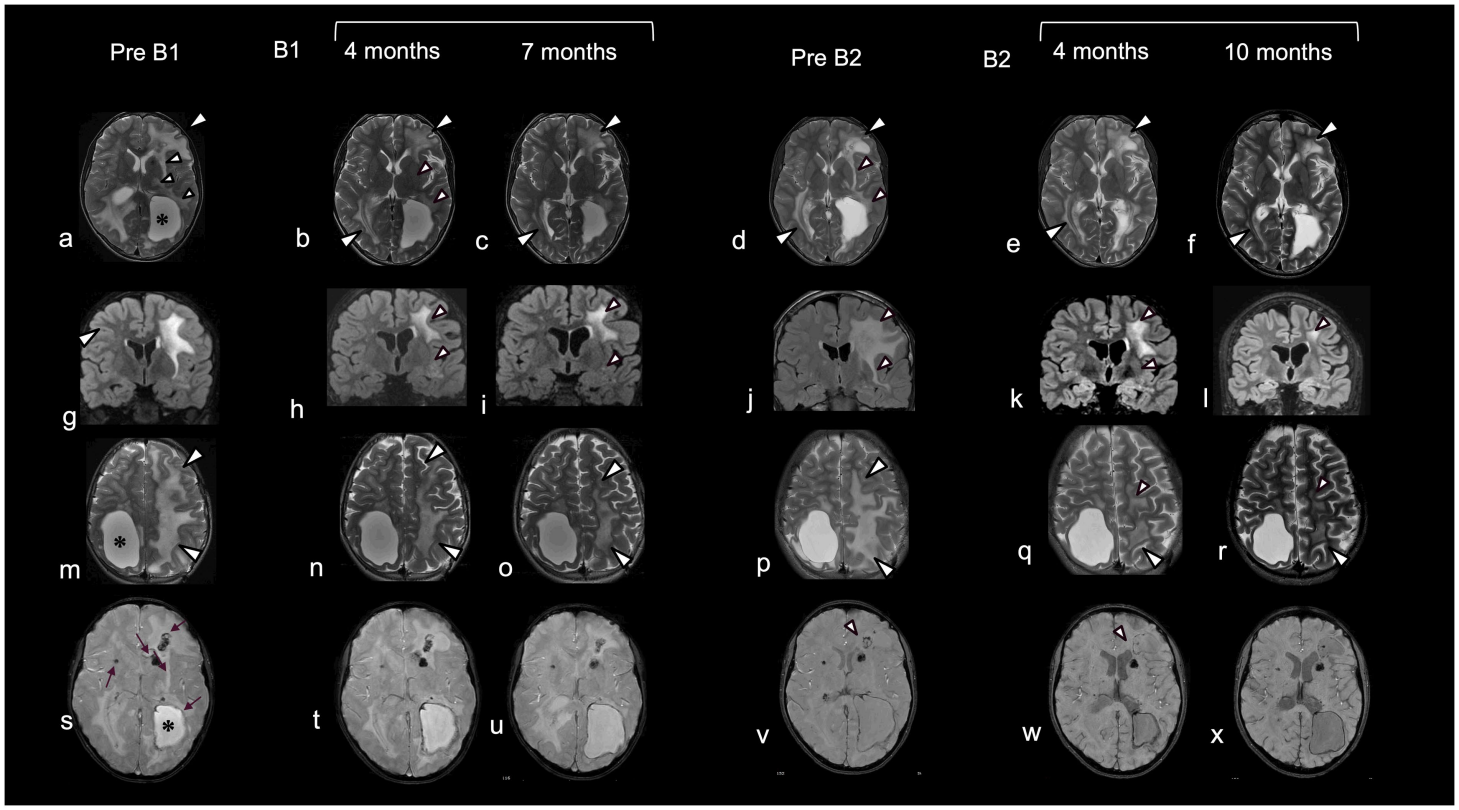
Neuroradiological data pre- and post-treatment with bevacizumab. Comparative brain MRI before and after the first and second cycle of treatment with bevacizumab. Before the initiation of treatment (pre B1) T2-weighted images (a,g,m) showed extensive and asymmetric hyperintensity of the periventricular and deep white matter, with involvement also of the juxtacortical U-fibers (a,m: white arrowheads) more extensive on the left, with lobar macrocysts (*) delimited by hypointensity in T2 Star Weighted ANgiography (SWAN) (s) due to calcium and hemosiderin deposition. Additional microcysts, microcalcifications and microdeposits of hemosiderin were present in the periventricular white matter, around the walls of the small cysts, and in the region of the putamen on the right and the caudate on the left (s: arrow). Four (b,h,n) and 7 months (c,i,o) after starting a first cycle of treatment there was a progressive reduction in the extent of the signal alteration, which first affected the white matter of the U fibers (b,c,n,o: white arrowheads), external capsular claustrum and posterior limb of the internal capsule and periventricular white matter (small white arrowheads). MRI images after discontinuation of treatment (pre B2) showed a reappearance of signal alterations in the same regions in which regression was observed (d,j,p vs a,g,m: white arrowheads). After initiation of the second treatment cycle, progressive improvement of signal alterations was observed in the same regions as after the first cycle (e,f,k,l). In addition, a reduction of signal hypointensities in SWAN sequences was observed in the frontal white matter on the left, around, and in proximity to, the cyst wall (v,w,x: arrowheads). Note the apparent decrease in abnormal white matter volume after treatment (c,o; f,r).
